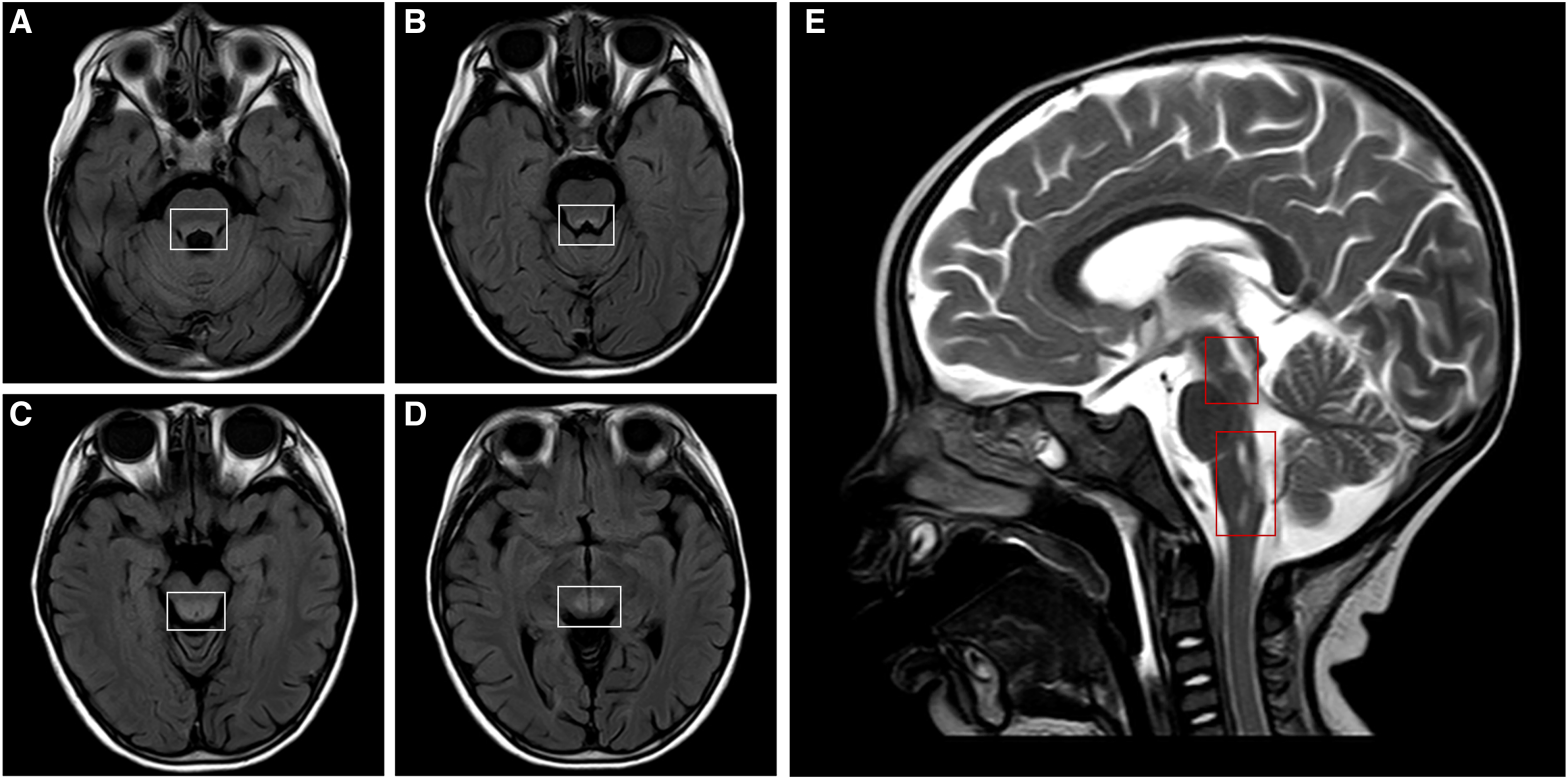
Cranial MRI of the patient. Cranial MRI shows bilateral symmetrical lesions of abnormal signal intensity around the third ventricle, pons, dorsal medulla oblongata, and in the gray matter around the dorsal aqueduct. Axial T2-FLAIR images (A–D, white frames) and a sagittal T2-weighted image (E, red frames) show the lesions. MRI, magnetic resonance imaging; FLAIR, fluid attenuated inversion recovery.
